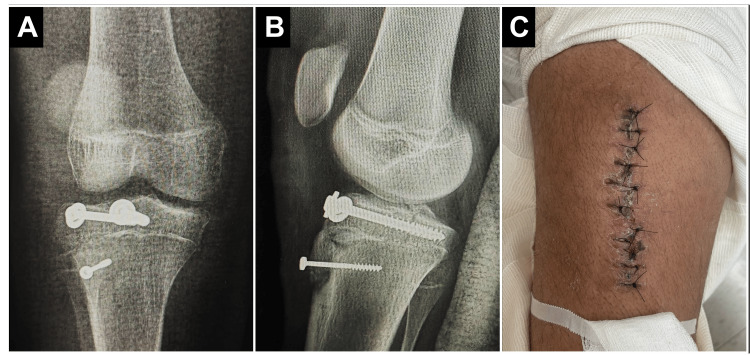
Postoperative outcomes following open reduction and internal fixation. (A) Anteroposterior and (B) lateral radiographs of the right knee showing anatomical reduction and stable fixation of the tibial tuberosity using partially threaded lag screws in bicortical configuration. (C) Clinical image of the right knee demonstrating satisfactory healing of the surgical wound with no signs of infection or dehiscence at early postoperative follow-up.
